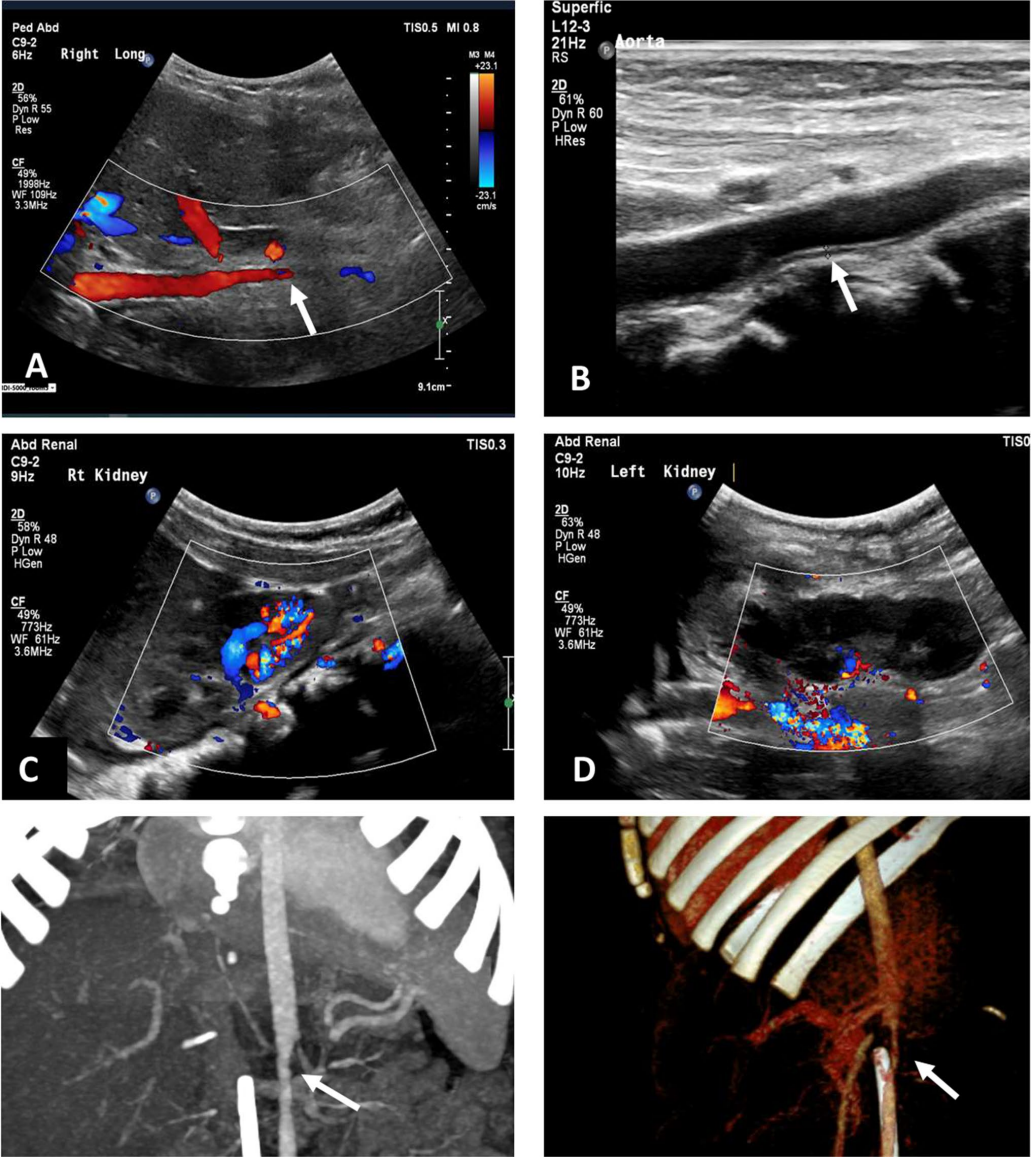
Doppler ultrasound of abdominal aorta and native kidneys at presentation. A Color Doppler ultrasound showing significant narrowing of the abdominal aorta lumen (arrow). B Gray-scale ultrasound of the abdominal aorta highlighting lamination of the vessel wall (arrow), suggestive of an inflammatory process. C Color Doppler ultrasound of the right kidney demonstrating vascularization limited to the lower pole. D Color Doppler ultrasound of the left kidney showing absent vascularization throughout the entire kidney. E CTA abdomen, coronal view showing segmental aortic wall thickening, enhancement, and stenosis (arrow)*.*
